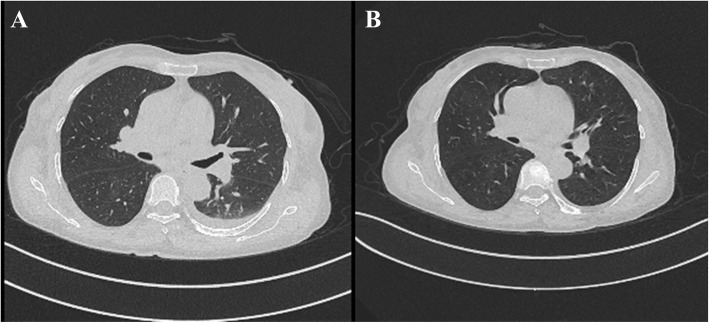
Computerized tomography (CT) images of the lungs. a, October 29; b, November 14
